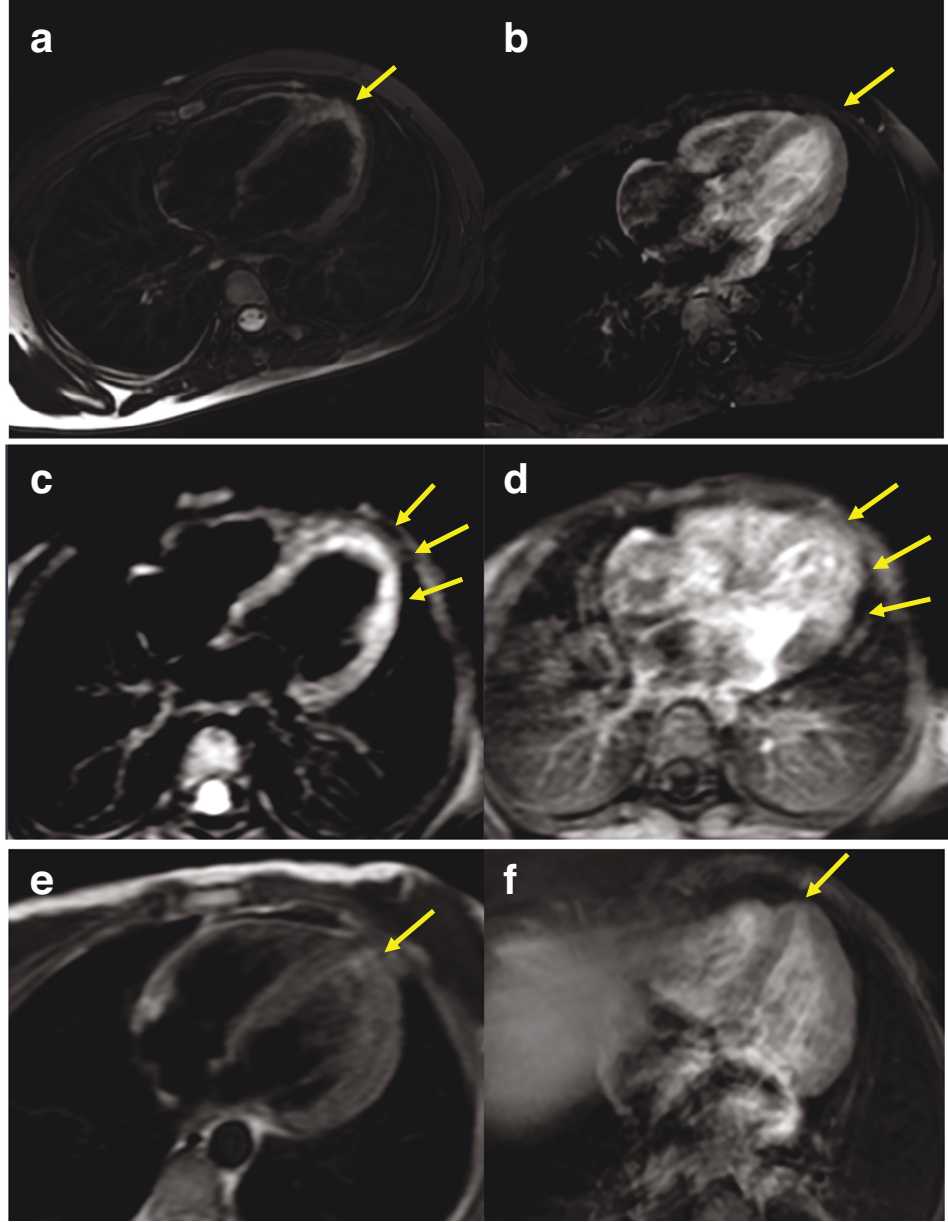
Cardiac magnetic resonance images of patients. Myocardial images of T2 scans (a, c, e) and late gadolinium enhancement T1 scans (b, d, f) on cardiac MRI. Cardiac MRI axial plane: (a, c, e) are T2 weighted fat saturated images and (b, d, f) are postcontrast late enhancement T1 weighted images. Myocardial edema is seen on segment 17 (apex) on images (a) and (e) and on segments 1, 2, 5, 6, 9, 10, 11, and 14 (basal anterior, basal anteroseptal, basal inferolateral, basal anterolateral, mid inferoseptal, mid inferior, mid inferolateral and apical septal) on the image (c). On postcontrast images (b) (d) and (f) contrast enhancement is seen on the segments parallel with myocardial edema areas on T2W-fat-saturated images.
